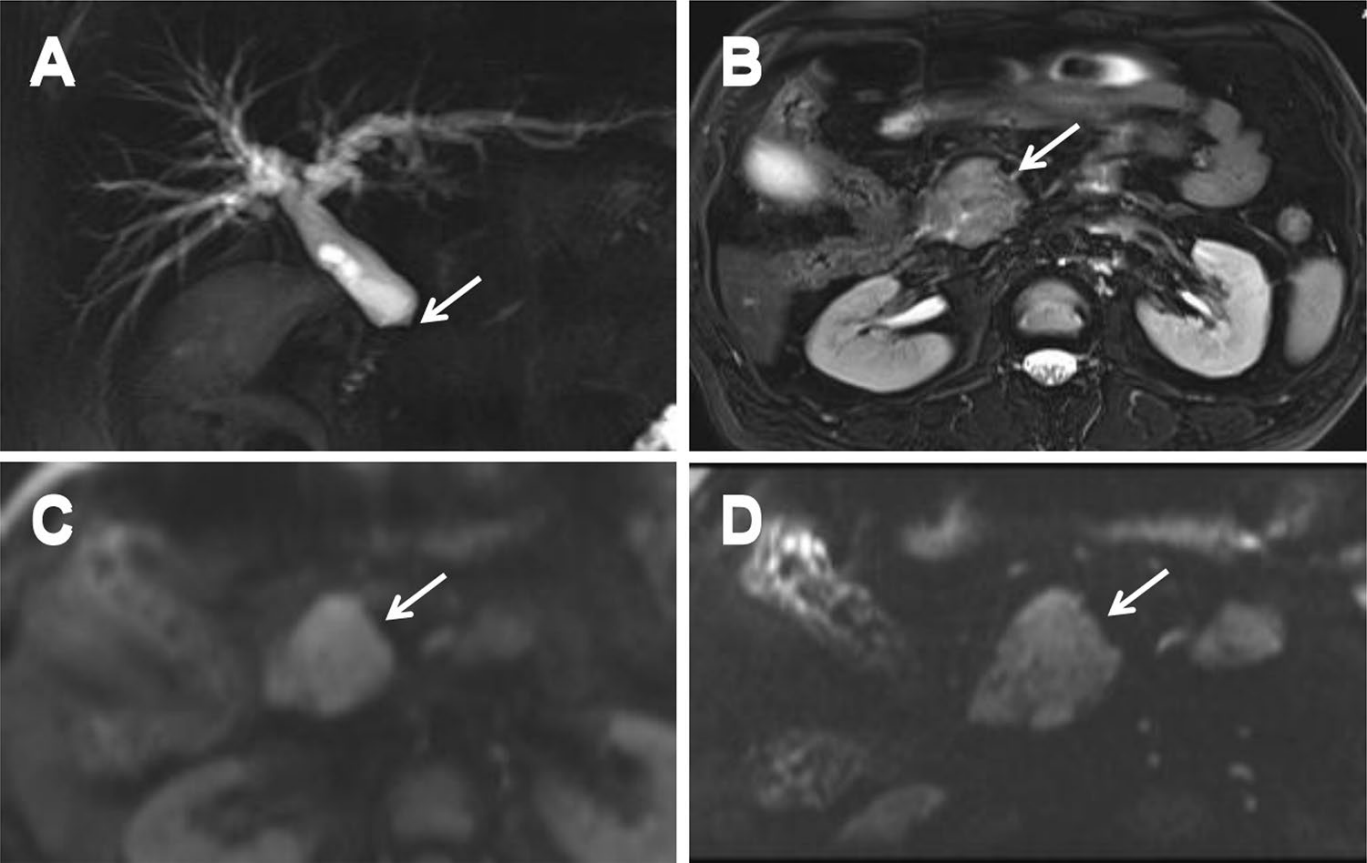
A 49-year-old man with autoimmune pancreatitis with a diameter of 37.3mm. A MRCP shows stenosis of the distal common bile duct (CBD). B T2-weighted MR image shows swelling of pancreas head. C c-EPI DWI shows no abnormality in the periampullary region. D z-EPI DWI also shows no abnormality in the periampullary region with better anatomic delineation
